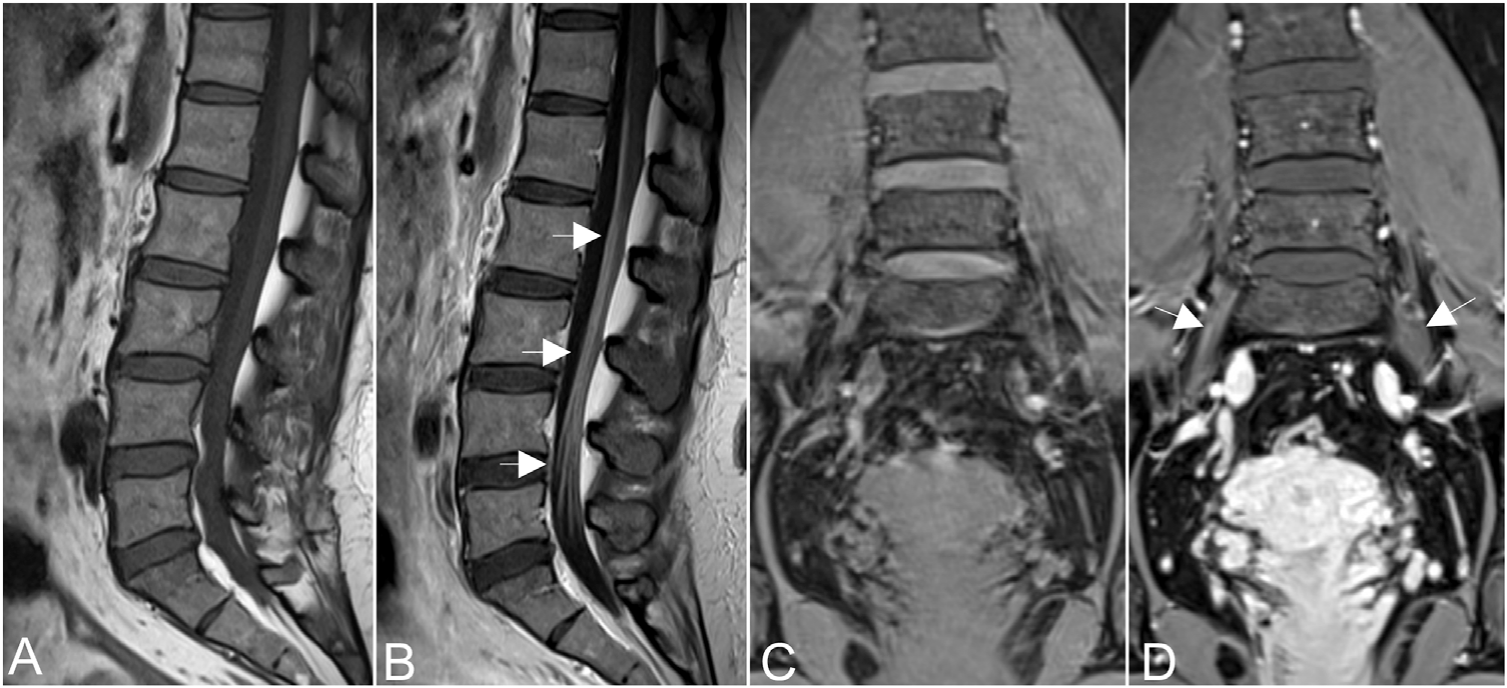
Obvious enhancement in the cauda equina [arrows in (A,B)], and mild enhancements were observed in the nerve roots of the hypertrophic plexus (arrows in (C,D)] on T1-weighted images after gadolinium administration.
